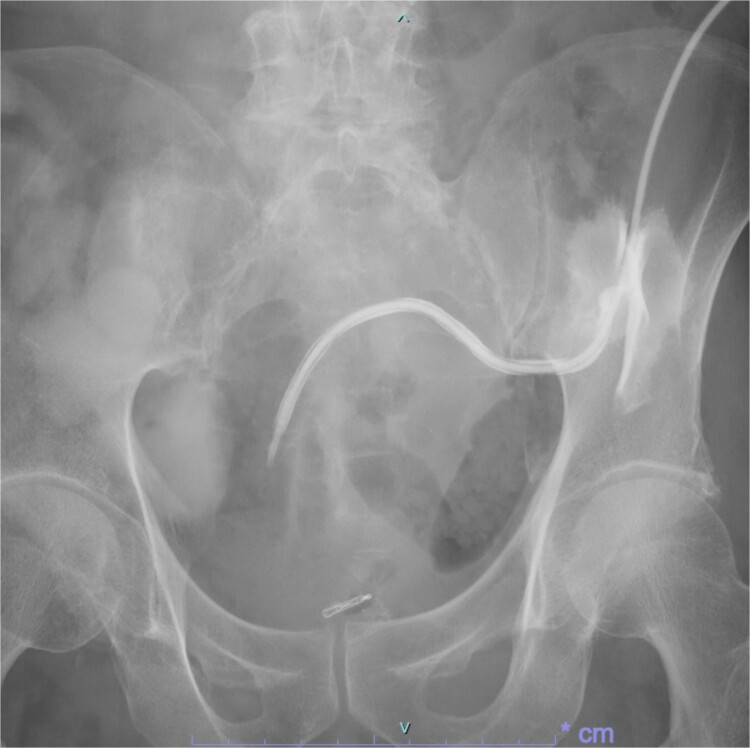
On day 15 after clipping, gastrografin injection under radiography reveals the fistula tends to recover.
